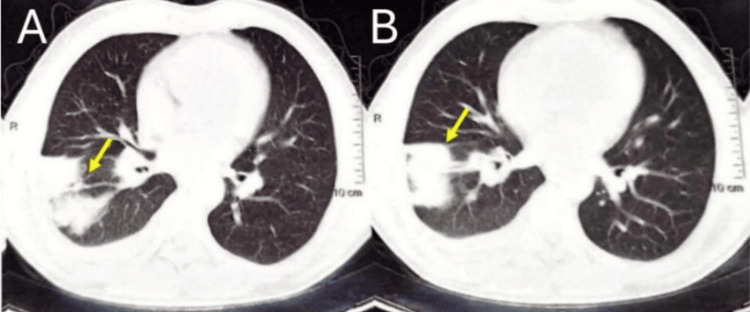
Axial chest CT images showing (A, B) right lower lobe consolidation with air bronchogram (arrows)
